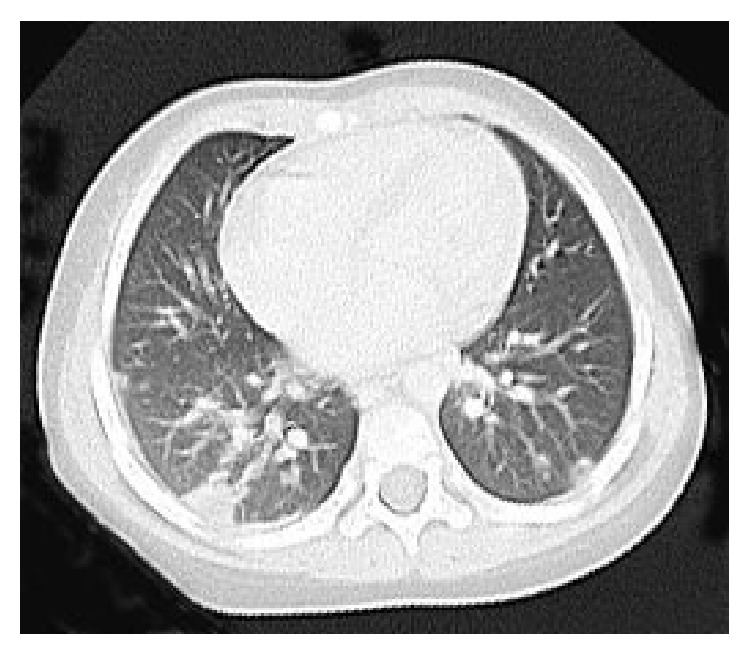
Computed tomography scan of the lungs without contrast showing necrotizing pneumonia with multiple nodules and pleural effusion on admission.
